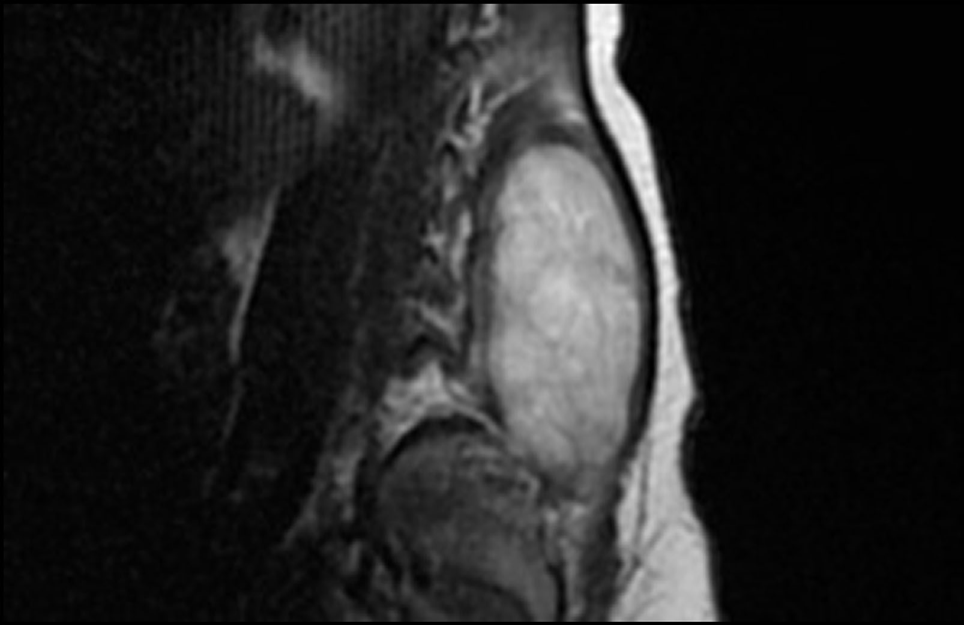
MRI before surgical excision (sagittal).
